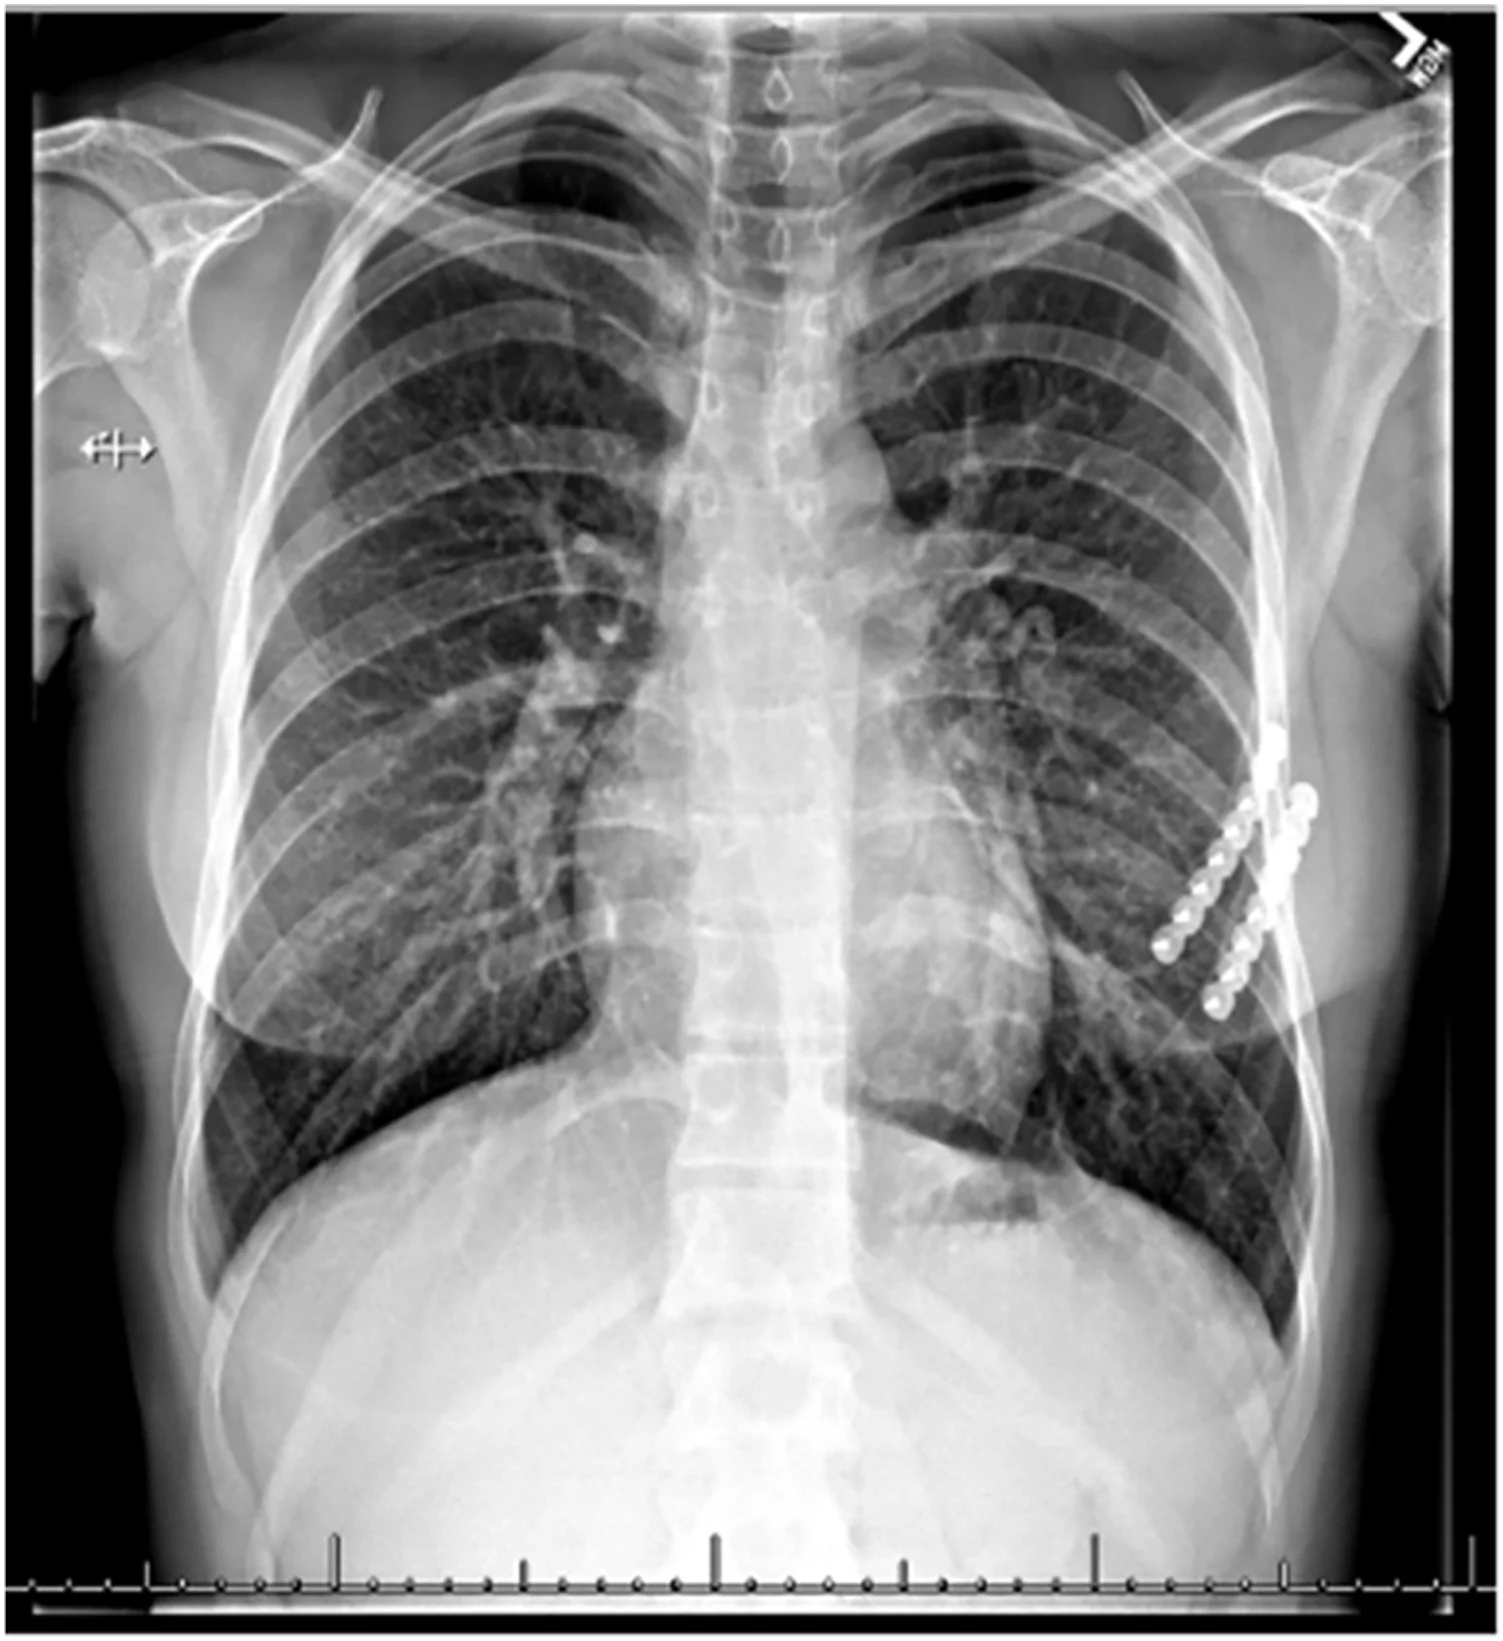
Postoperative chest radiograph of rib plates.
